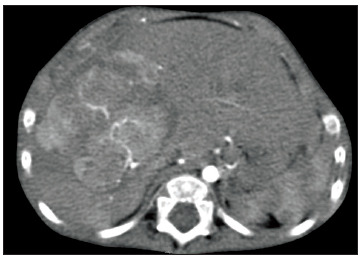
Computed tomography (CT) scan of the abdomen. A space-occupying lesion is visible in the liver, located between the middle and right hepatic veins (segment VIII).
